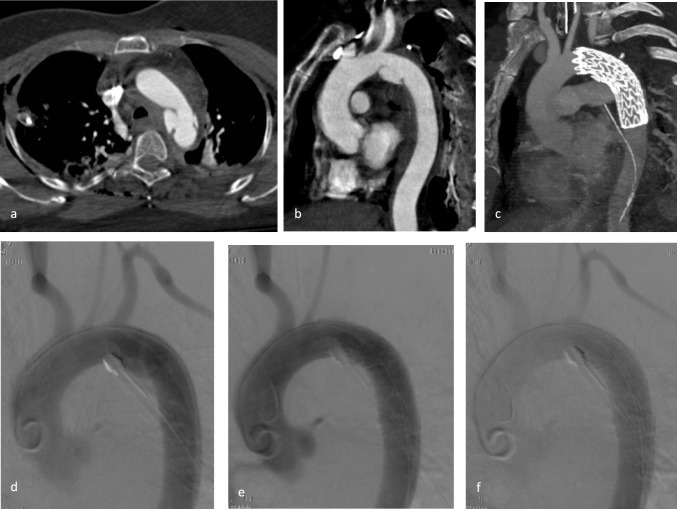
Grade IV aortic isthmic lesion treated with Gore C-TAG 26 × 100 mm. a b Preoperative axial and para-coronal CTA and d aortography; c postoperative para-coronal CTA and e f aortography: correct positioning and patency of endoprostheses, no complications; LSA dulling from retrograde revascularization
